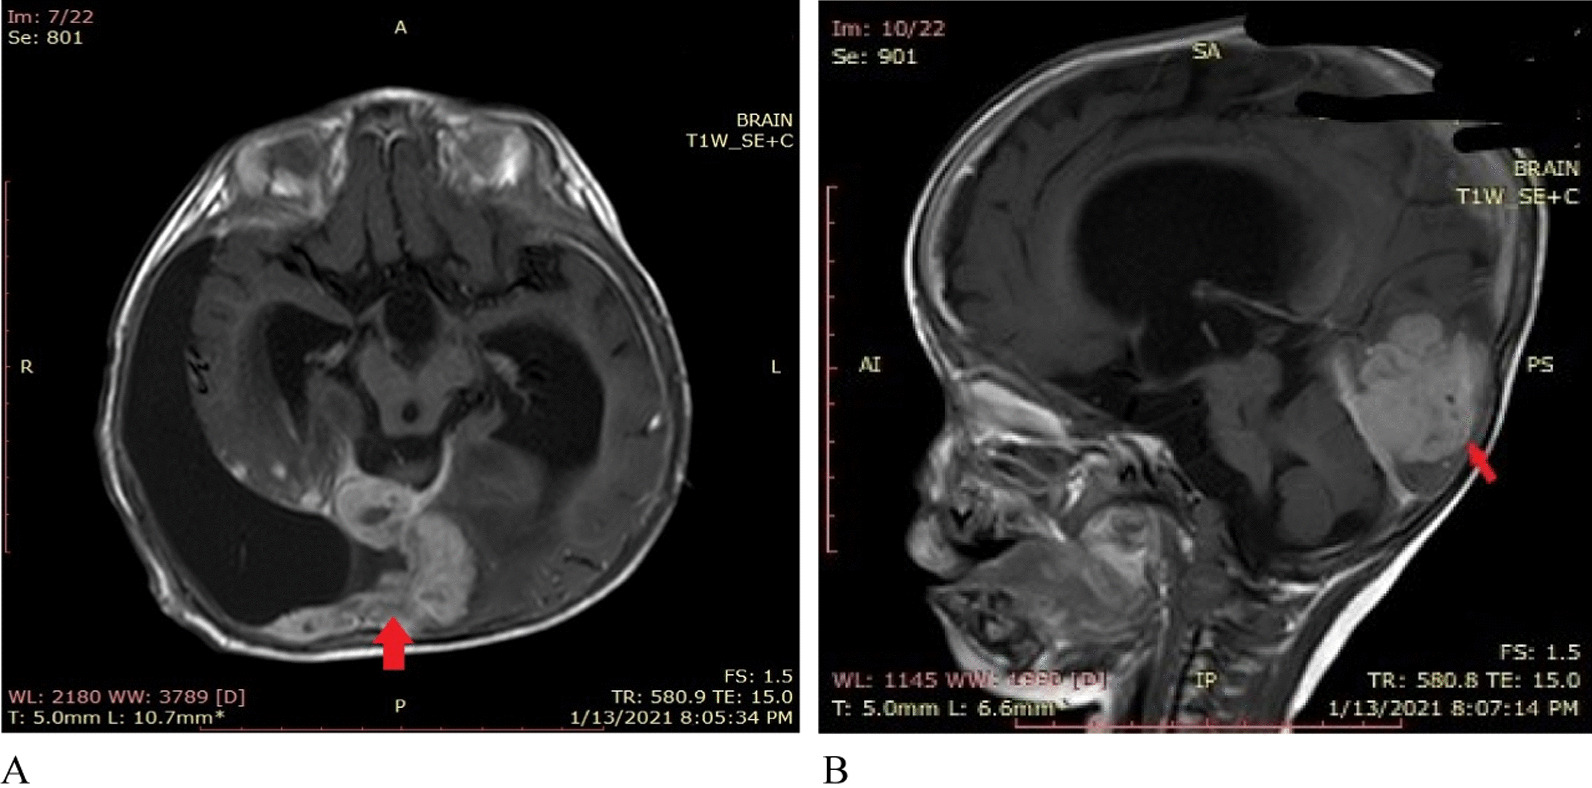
A, B Two months postoperative MRI with contrast. Axial and sagittal images show a homogeneously enhancing dural based mass (4.2 × 2.6 cm) (red arrow) adherent to tentorium cerebelli and posterior interhemispheric fissure
